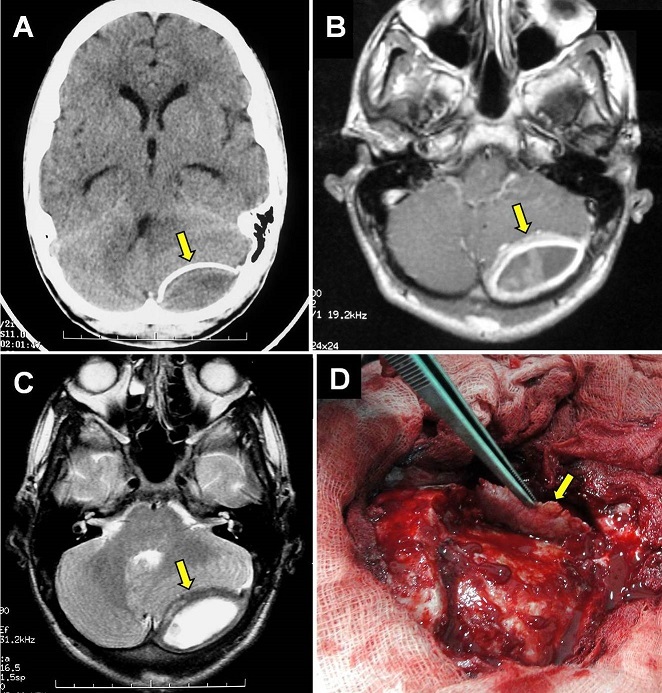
Axial CT-scan. (A): revealing a left posterior fossa chronic extradural biconvex lesion with ossified wall (arrow). MRI: Axial T1-weighted image after gadolinium injection; (B): T2-weighted image; (C): fluid-liquid lesion with homogeneous ring enhancement and significant cerebellar compression; (D): operative view demonstrating the ossified wall (arrow) after superficial dissection from the duramater
